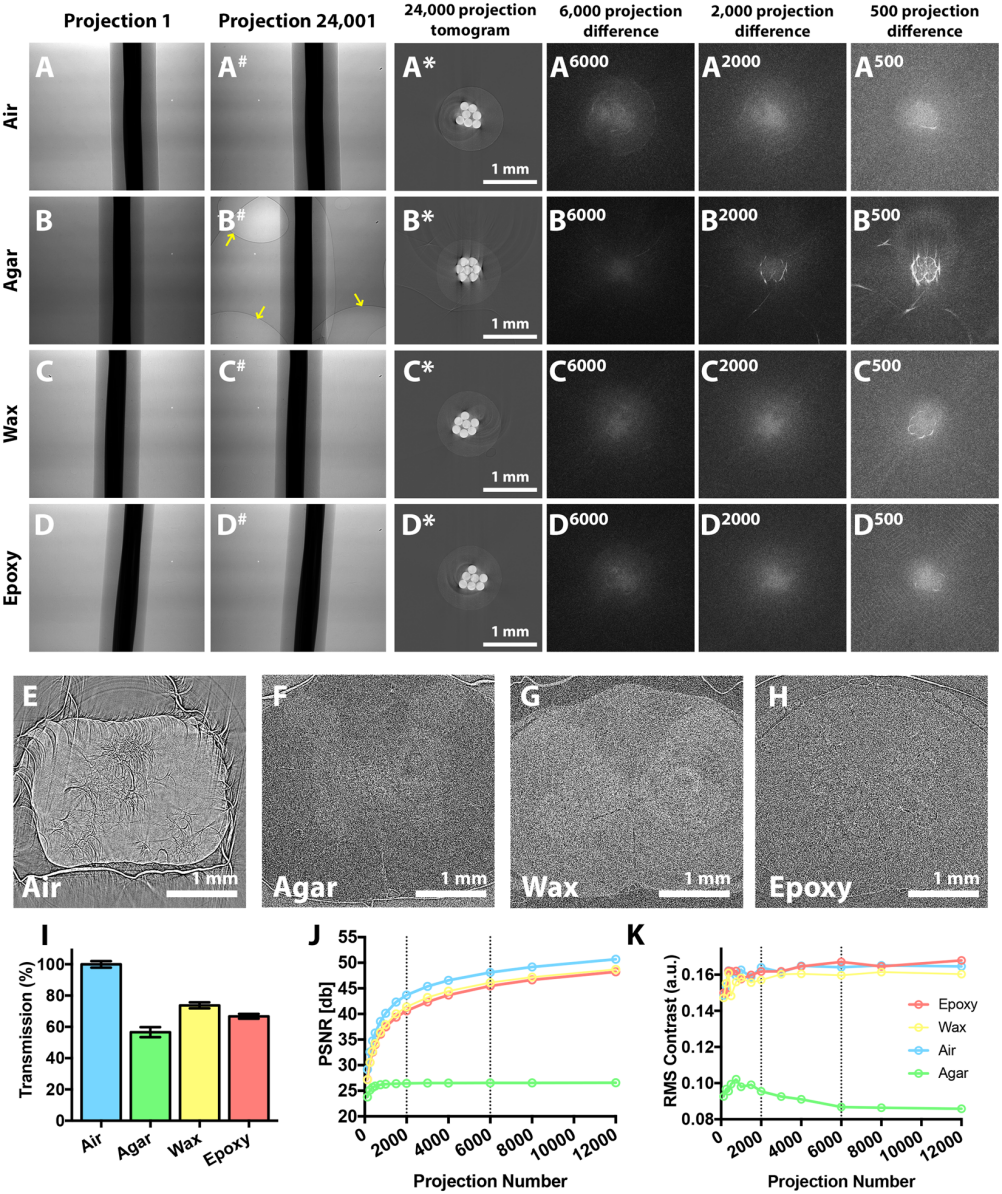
Testing embedding materials and optimising projection number. (A–D) Standard comparison samples made from embedding a piece of plastic-insulated wire containing seven drawn copper pieces in air, agar, wax and epoxy. These were subjected to an ‘over-projected’ ~40-minute scan (24,001 projections, 80 ms exposure). Comparing the (A–D) first and (A^#^,B^#^,C^#^,D^#^) last (24,001^st^) projection of each scan showed little deviation in air, wax and epoxy samples, confirming their stability during X-ray exposure. However, during agar scanning (B), expanding air bubbles (yellow arrows) shunted the wire piece. (A*,B*,C*,D*) Tomographic reconstructions were compromised in the (B*) agar-embedded sample by the sample being shifted by these expanding air bubbles. Difference maps between full-projection tomograms and tomograms reconstructed from 6,000 (A^6000^,B^6000^,C^6000^,D^6000^) 2,000 (A^2000^,B^2000^,C^2000^,D^2000^) and 500 (A^500^,B^500^,C^500^,D^500^) projection subsets. (E–H) 6,001-projection scans of a piece of unstained spinal cord embedded in each medium (propagation distance 20 mm) show that ‘air embedding’ does not adequately stabilise spinal cord samples. (I) X-ray transmission, assessed by comparing ten 100 × 100 pixel ‘background’ (embedding material only) regions in images of each embedding material to a flat-field image. Wax and epoxy absorbed ~30% of transmitted X-rays, with ~10% more absorbed by agar (mean ± standard error of the mean). (J) The peak signal to noise ratio (PSNR) measured in decibels [db] confirms that increasing projections reduces background noise, with ~85% saturation reached with 6,000 projections for wax. (K) The root mean square (RMS) contrast shows that increasing projections improves contrast, but this quickly saturates beyond ~2,000 projections.
